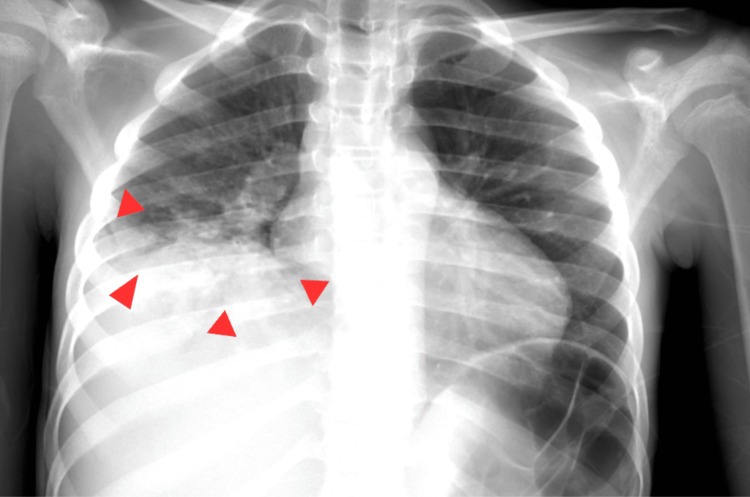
AP chest radiograph demonstrates a right-sided loculated pleural collection with associated pleural thickening and adjacent compressive atelectasis, consistent with empyema
